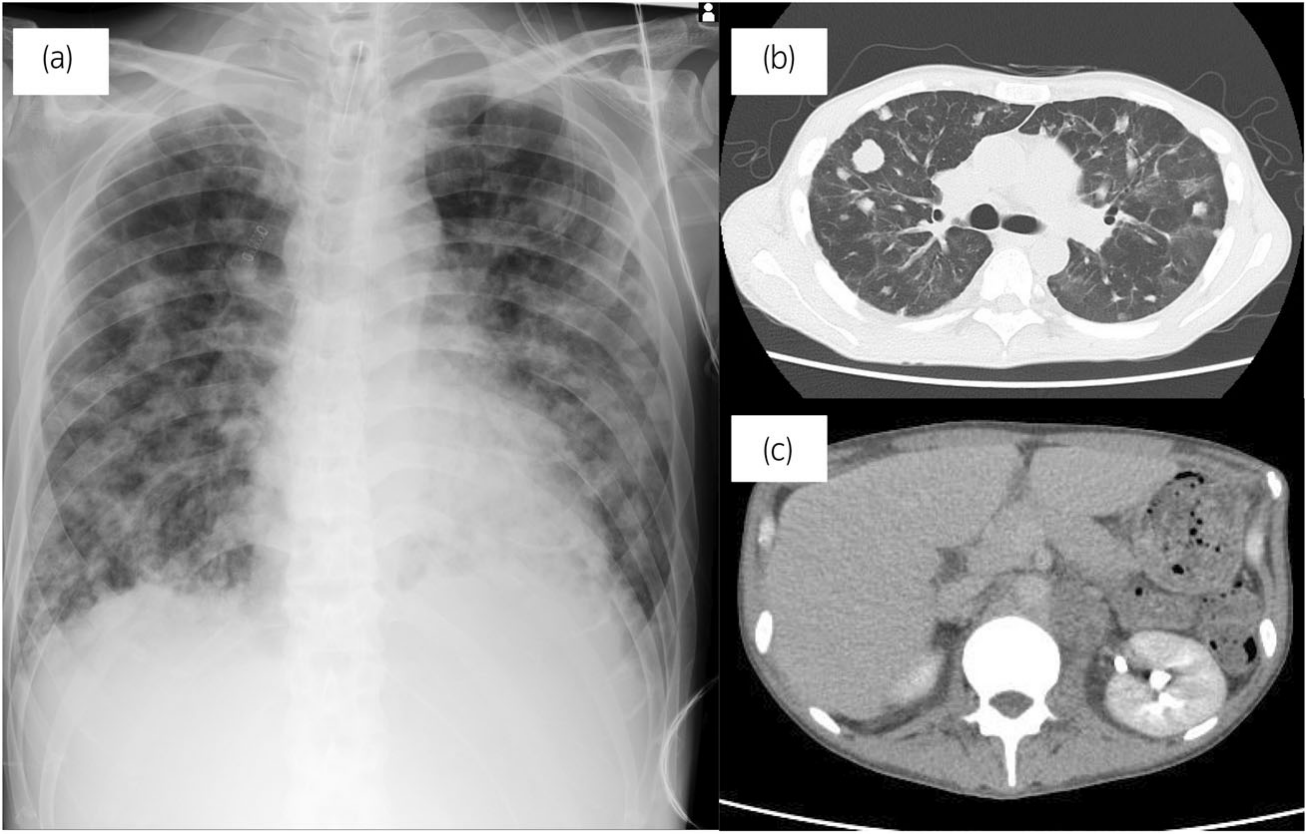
Image at the discharge from ICU. Chest X‐ray (a) and CT (b) Infiltration shadows in the lung fields decreased, compared with those before chemotherapy. (c) Abdominal contrast‐enhanced CT scan showed reduction of retroperitoneal lymph nodes.
